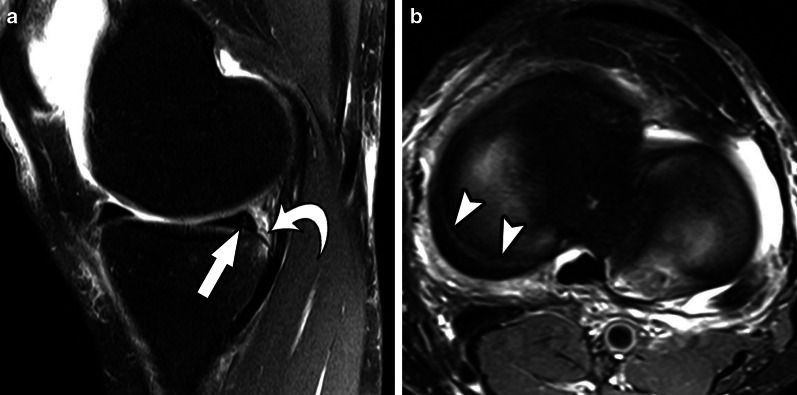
Type 3B meniscal ramp lesion MRI Case. Sagittal (a) and axial (b) T2-weighetd fat-suppressed images show peripheral partial vertical tear affecting the tibial surface of the posterior horn of medial meniscus (arrow), along with meniscotibial ligament tear (curved arrow) and bone bruise; extension of vertical tear is seen in (b) (arrowheads)
